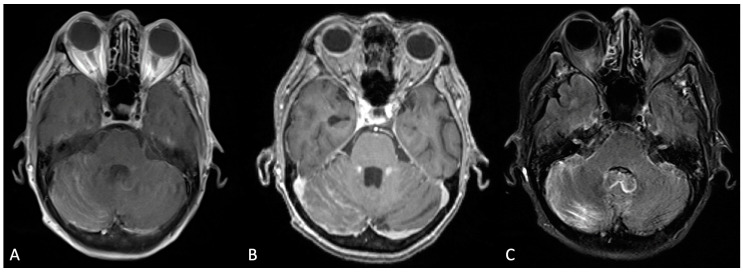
Bilateral cerebellar folia hyperenhancement, more prominent on the right, was observed in the post-contrast T1-weighted Turbo Spin Echo (TSE) sequence (A); the T1-weighted Multi-Planar Reconstruction (MPR) sequence (B); and the T2-weighted Turbo Inversion Recovery Magnitude Fat Suppressed (TIRM-FS) sequence (C).
